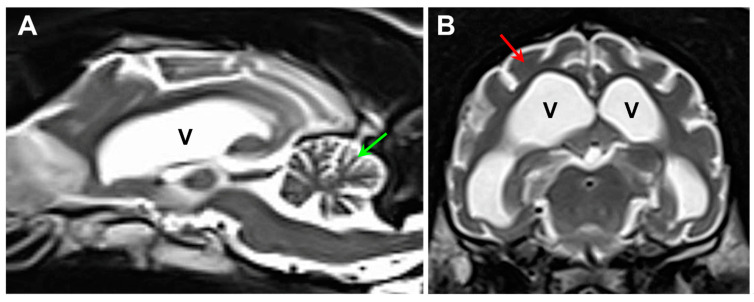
Sagittal (A) and axial (B) T2-weighted MR images of the proband’s brain. The ventricular system (V) was dramatically enlarged, and there was a pronounced shrinkage of the parenchyma of both the cerebellum (green arrow in (A)) and the cerebral cortex (red arrow in (B)).
